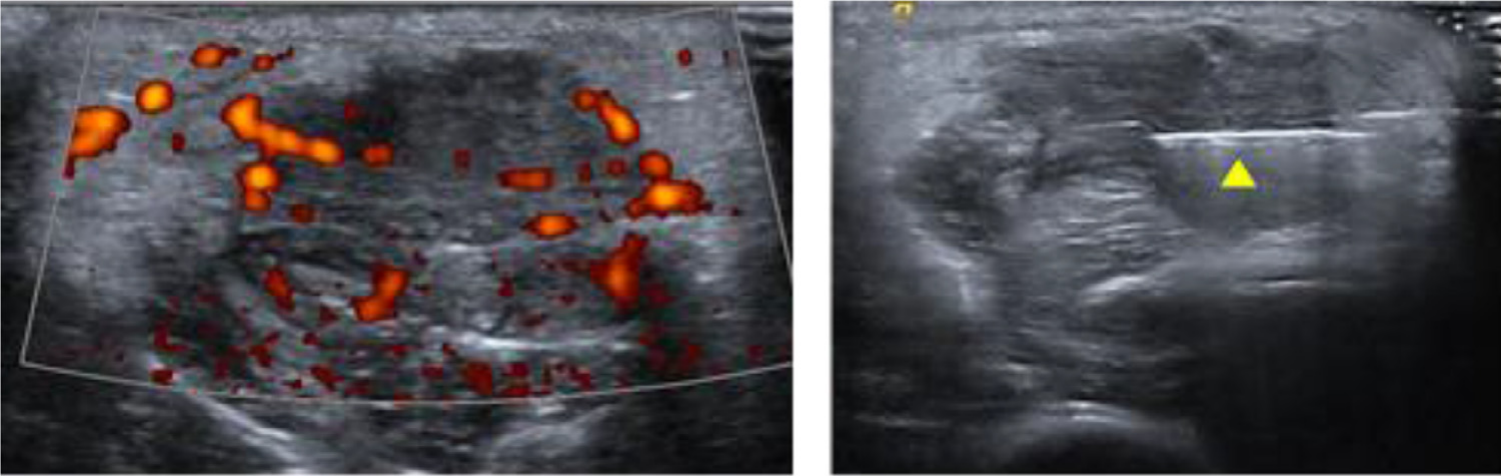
(A) Short axis color Doppler US showing solid hyper vascular soft tissue mass involving the deltoid muscle belly. (B) US-guided Temno needle (yellow arrowhead) biopsy of the mass.
